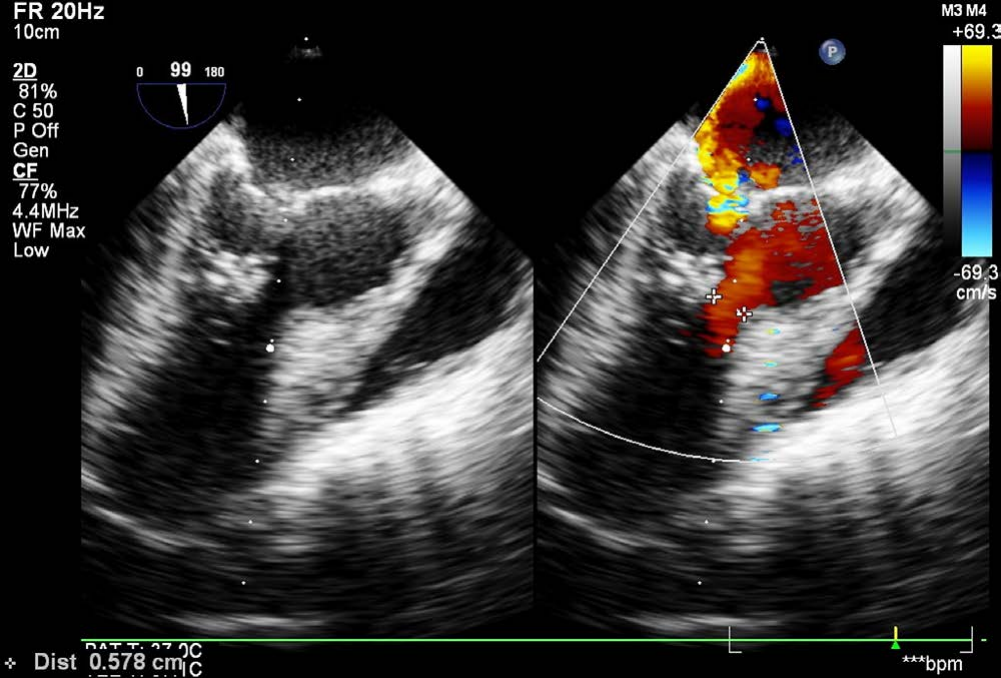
Cardiac ultrasound after surgery.
